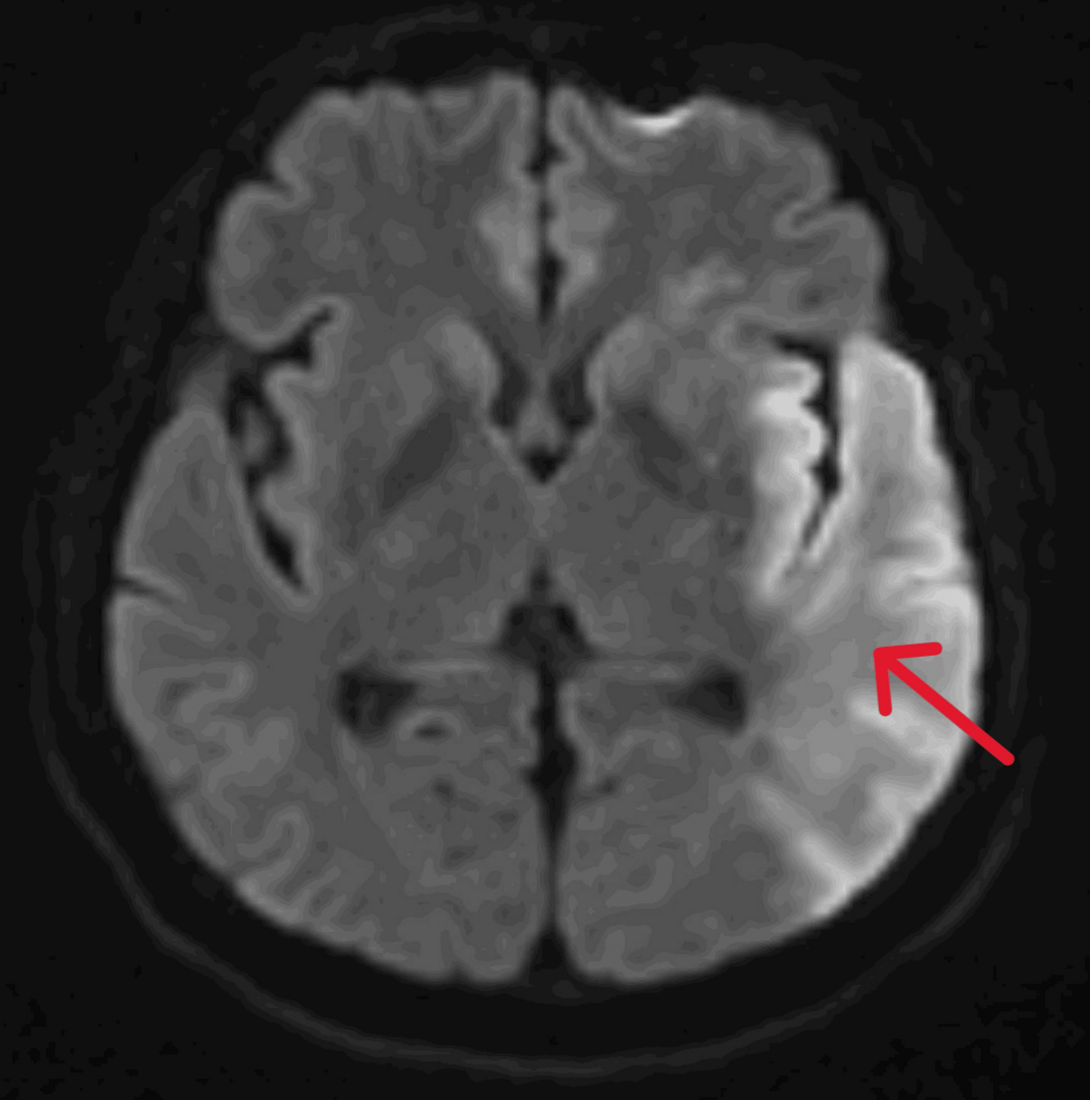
An MRI of the brain showing acute ischemic infarction of the left middle cerebral artery distribution. MRI: magnetic resonance imaging
